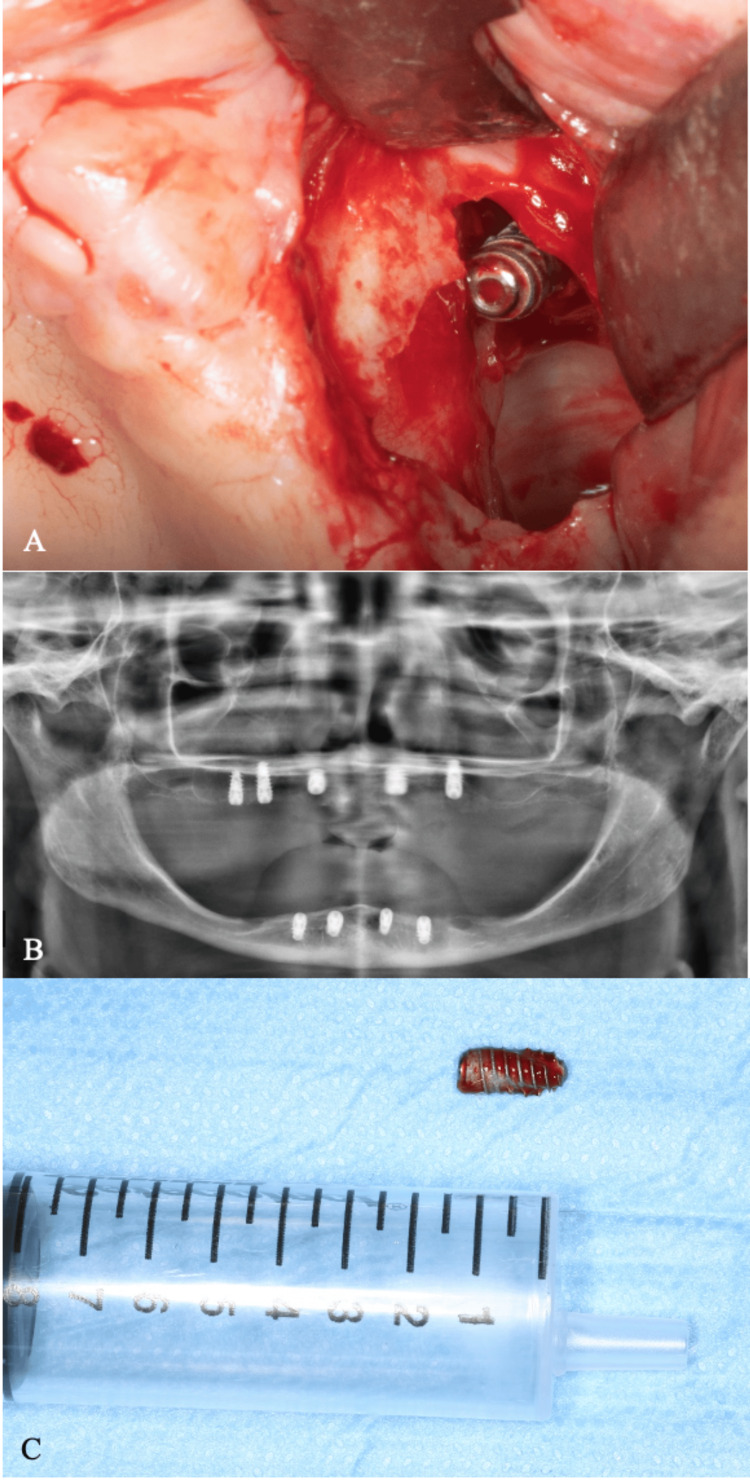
Retrieval of the implant. Intraoral view showing the implant within the maxillary sinus prior to retrieval (A). Postoperative panoramic radiograph taken after retrieval of the implant (B). Clinical photograph of the removed implant (C)
